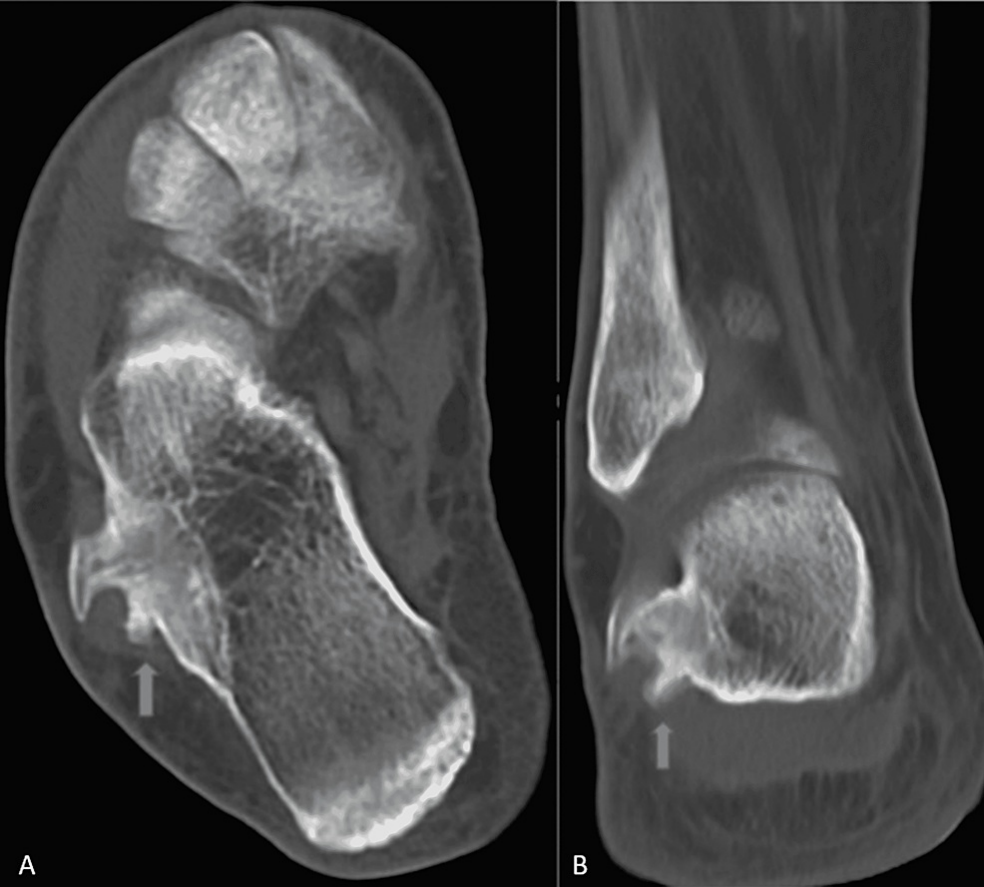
Bony overgrowth of calcaneal peroneal tubercle on CT scan. A: axial plane; B: coronal plane.
